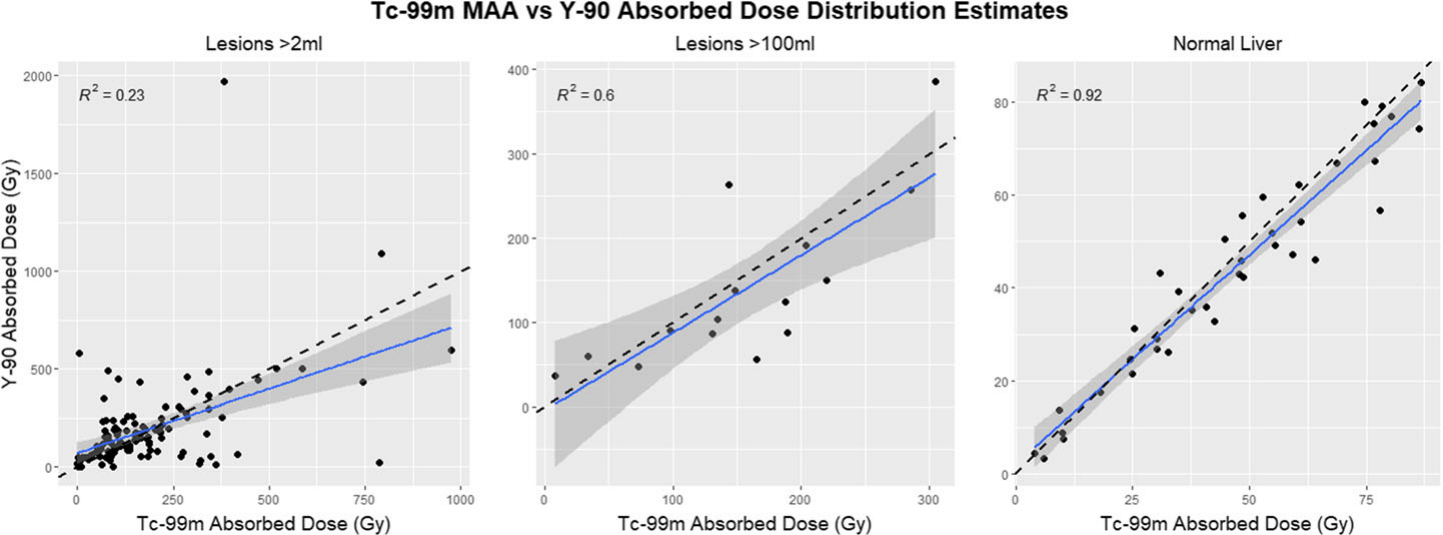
Comparison between absorbed dose estimates for ^99m^Tc-MAA SPECT and ^90^Y PET. The dashed line denotes the identity line while the solid line shows the current linear fit
